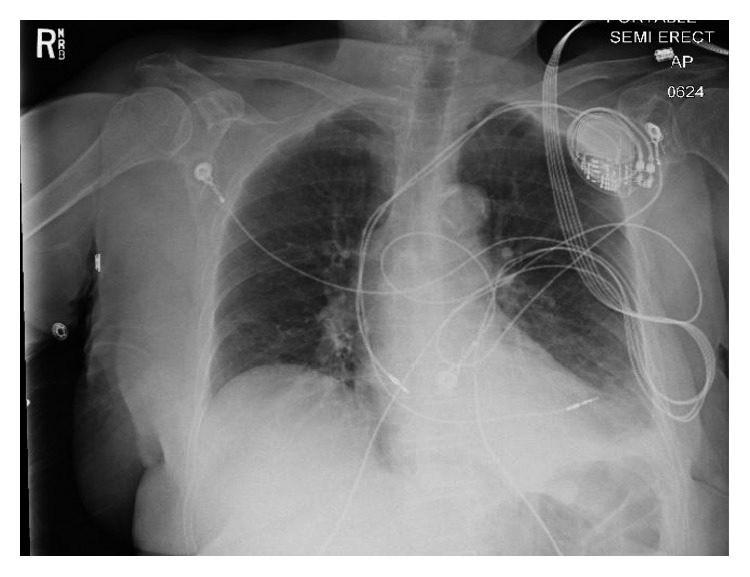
A chest X-ray of the patient on presentation revealing the right ventricular lead overlying the left.
